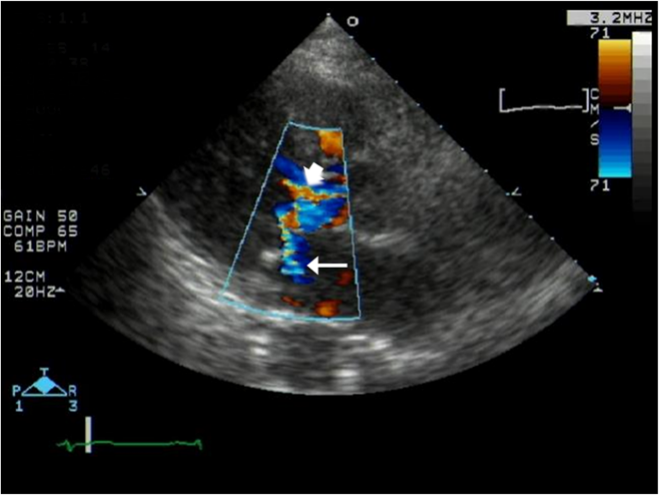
Bedside transthoracic echocardiography with color-flow Doppler mapping in the post-anesthesia care unit after sublingular nitrate (left parasternal long axis view). Significant mitral regurgitation jet in the posterior direction (arrow)and mosaic pattern left ventricular outflow (arrow head) appeared.
